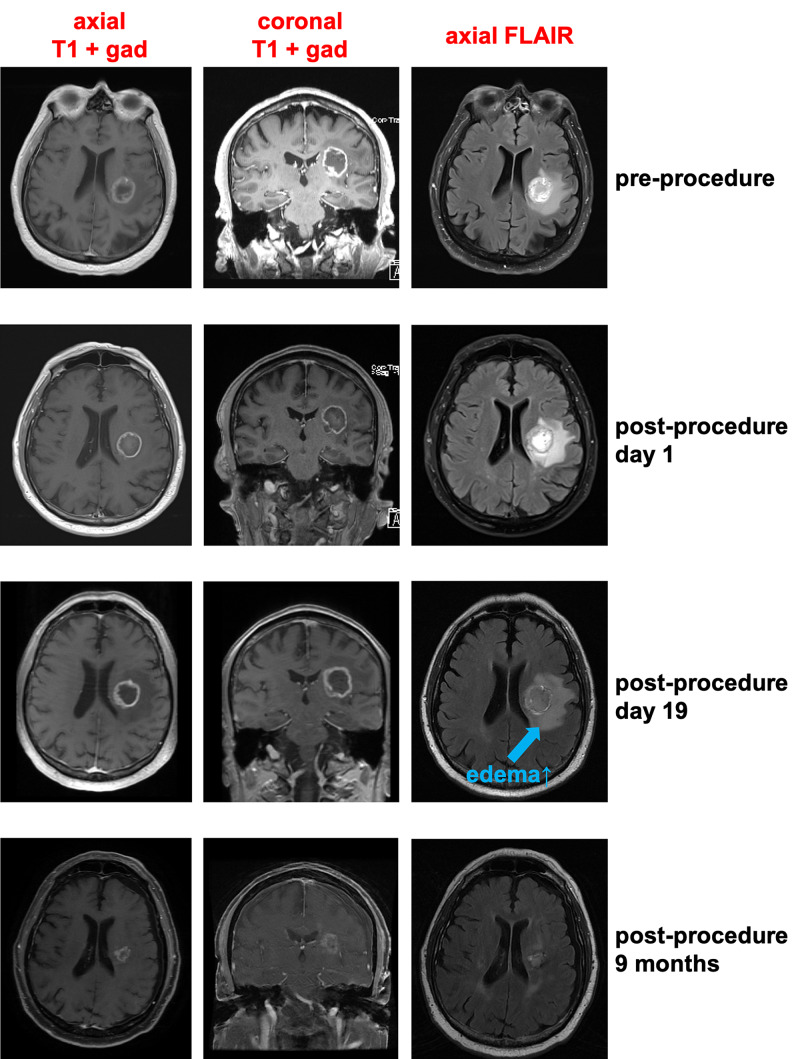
Delayed edema following laser ablation of tumors. We show relevant MRI images of patient 1, who underwent laser ablation of a left subinsular IDH wild-type GBM. Note that the peritumoral FLAIR signal is modestly increased in the MRI performed 19 days after the ablation. This was associated with worsening right hemiparesis that responded to dexamethasone therapy. Soon thereafter, the patient was treated with adjuvant chemoradiotherapy. Also note that nine months later, the enhancing tumor volume and peritumoral FLAIR signal have decreased significantly. That patient was being treated with bevacizumab at that time.
